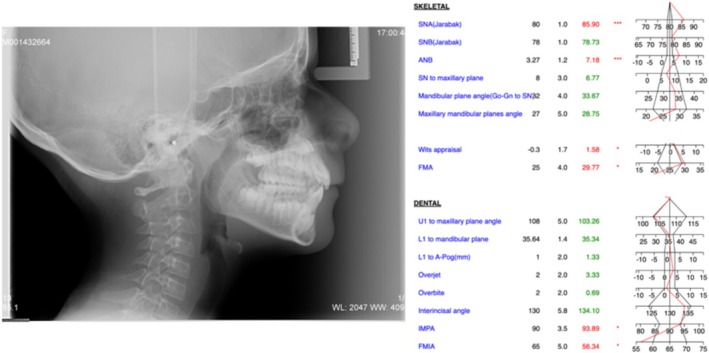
Lateral cephalometric radiograph and cephalometric analysis.
